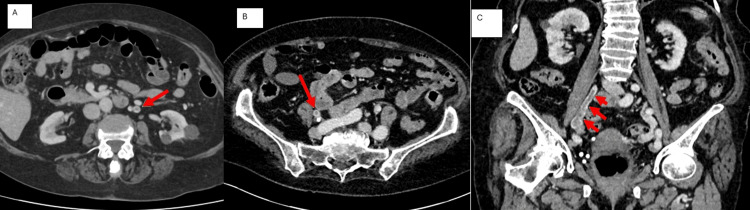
CT scan of the abdomen and pelvis with contrast (axial view) showing (A) left ovarian vein thrombosis (red arrow) and (B) right ovarian vein thrombosis (red arrow). (C) CT scan of the abdomen and pelvis with contrast (coronal view) showing right ovarian vein thrombosis (red arrow)
